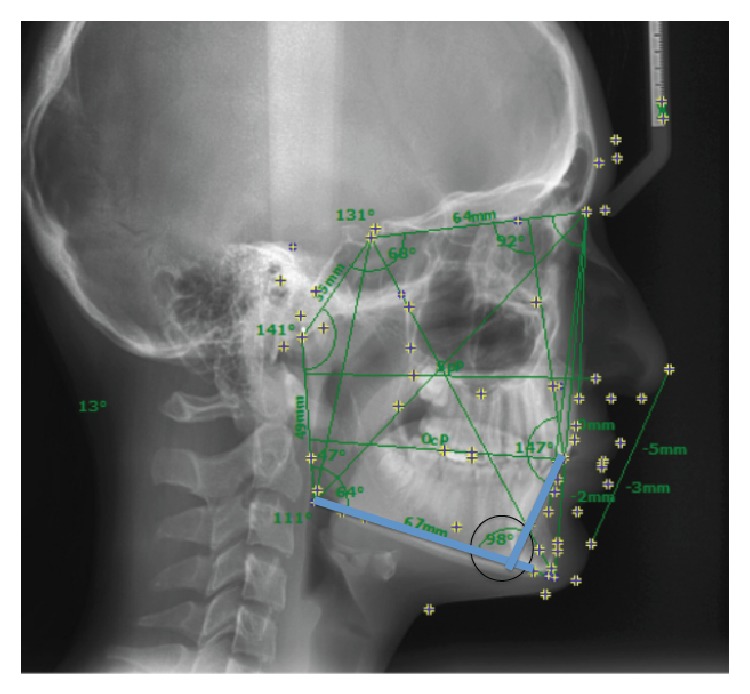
The degree of lower incisor inclination was measured by calculating the angle between mandibular plane (MP) and the long axis of most forward lower incisor on lateral cephalometric radiographs.
